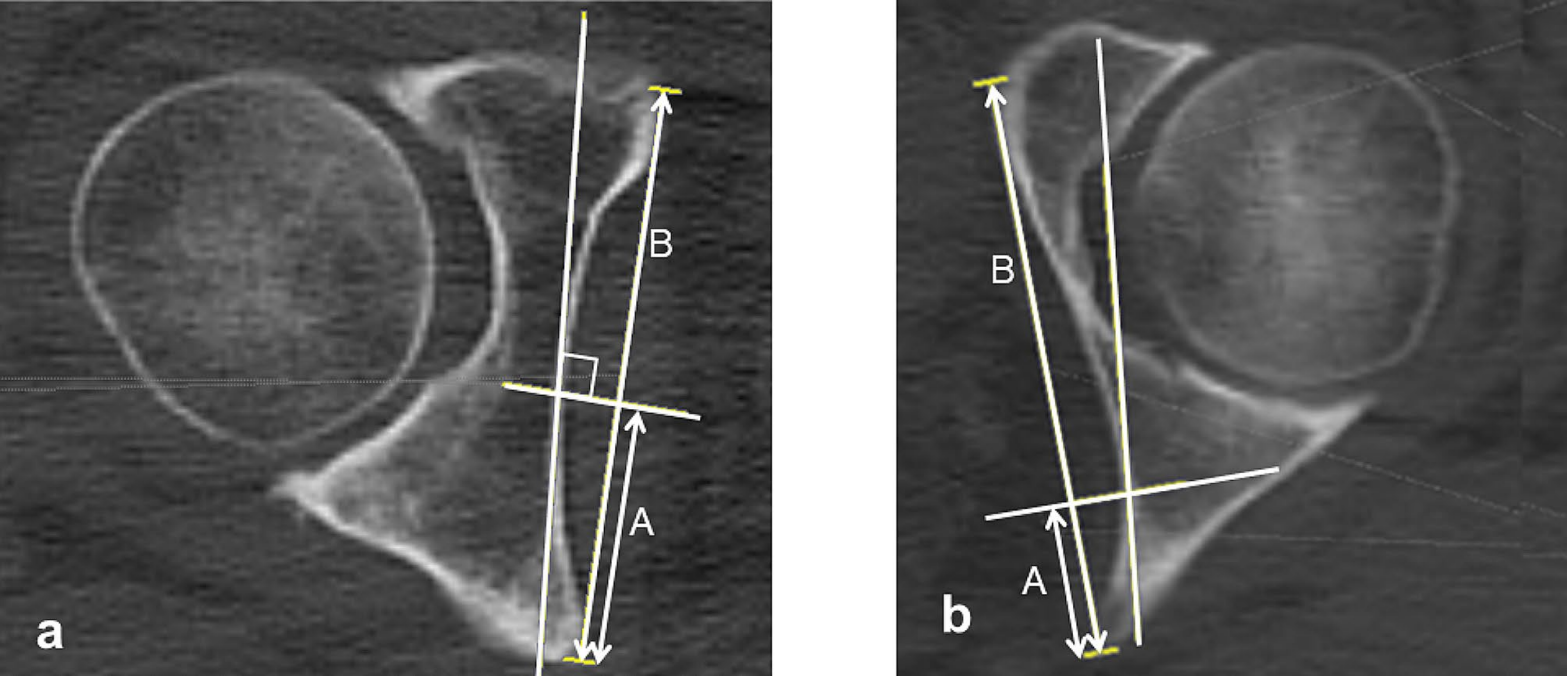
Measurement of the posterior ratio of the ilioischial line point on the axial CT slice The posterior ratio of the ilioischial line point on the axial CT slice was obtained by dividing the length from the center point of the cortical bone, indicating the ilioischial line to the posteromedial margin of the ischium (A), by the length from the anteromedial pubis margin to the posteromedial ischial margin (B). a A coxa profunda-negative case. b A coxa profunda-positive case. CT, computed tomography
